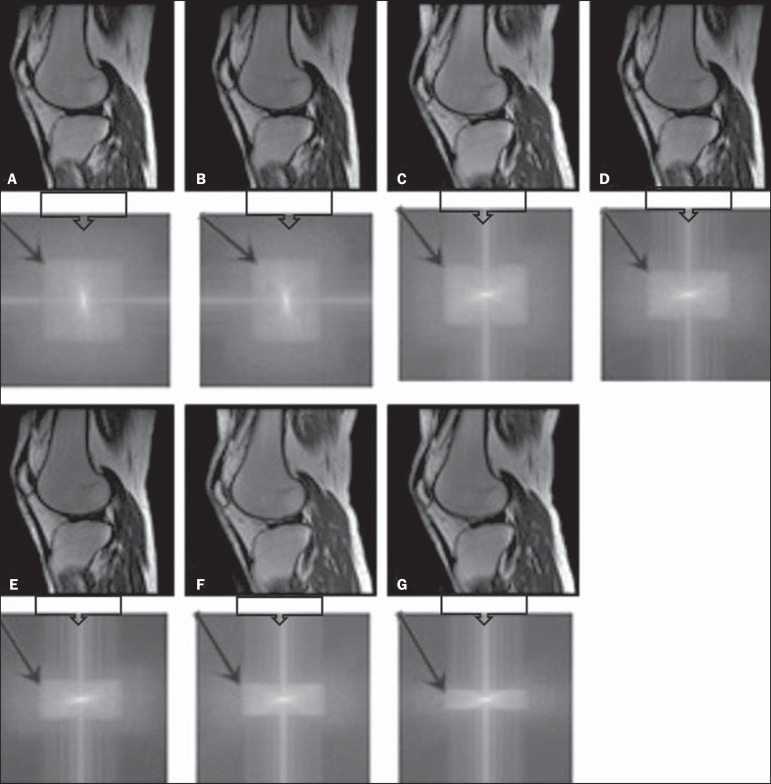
T1-weighted magnetic resonance imaging scans with the following ScP
